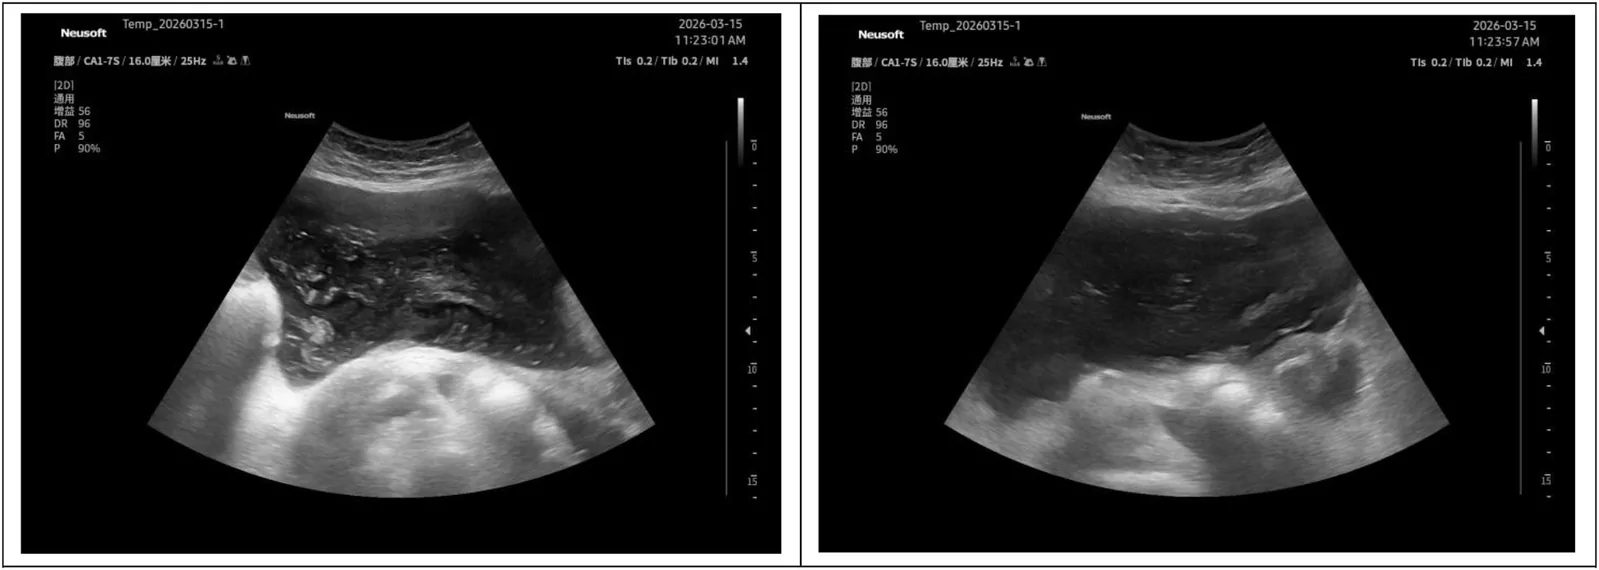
Ultrasound: The abdominopelvic effusion shows poor acoustic transmission, with multiple mucin particles and fibrous septations visible internally.
